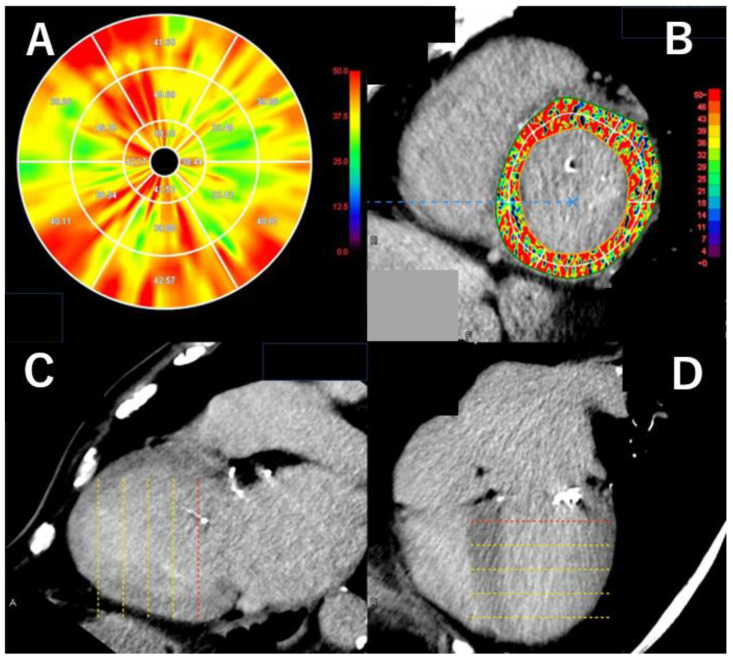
Extracellular volume analysis of left ventricular myocardium in a case with severe aortic valve stenosis. Extracellular volume (ECV) of left ventricular myocardium (LVM) on computed tomography (CT) in this patient with severe aortic valve (AV) stenosis was 39.37% (A), and this patient was admitted because of congestive heart failure 12 months after the transcatheter AV implantation(TAVI). ECV was measured using a specific software (Ziostation 2, Ziosoft, Tokyo, Japan). Short axial, 2-chamber, and 4-chamber views of late enhancement LVM were shown in Figure (B–D). The dotted lines over the LV in the long-axis images (C,D), indicate the slices of the short-axial images used for analysis (The red dotted line indicates the currently displayed short axis image) (B).
